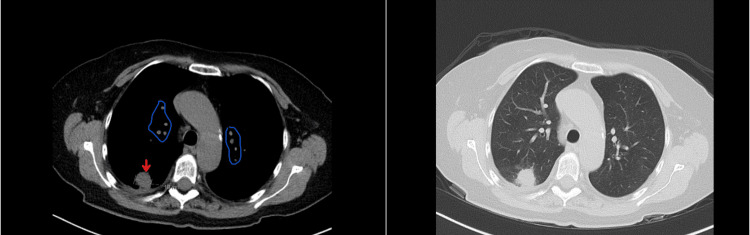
CT chest showing 18 mm nodule in right lower lobe (red arrow) and multiple smaller nodules bilaterally (encircled in blue) (left), high-density CT version of the same scan (right)
